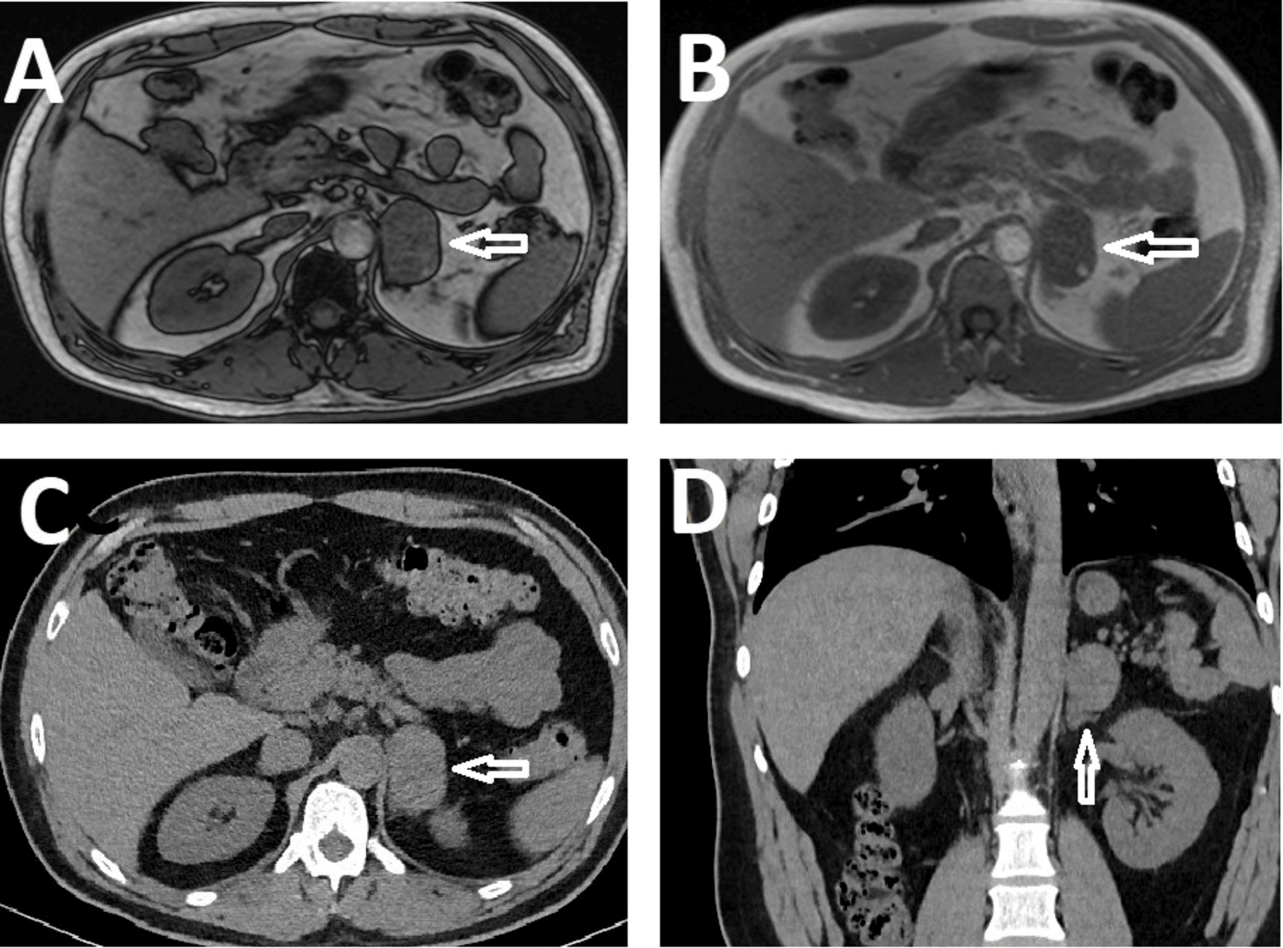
Adrenal imaging A) In-phase axial magnetic resonance imaging (MRI) image of a 4.9 x 3.2 x 4.0 cm ovoid heterogeneous left adrenal mass with internal soft tissue (hollow white arrow). B) Axial MRI image showing no dropout of signal intensity on the opposed, out-of-phase sequence (hollow white arrow). C) Axial computed tomography (CT) scan image. D) A coronal CT scan image of dense, lipid-poor, left adrenal mass with radiodensity measuring 32 Hounsfield units (hollow white arrow).
